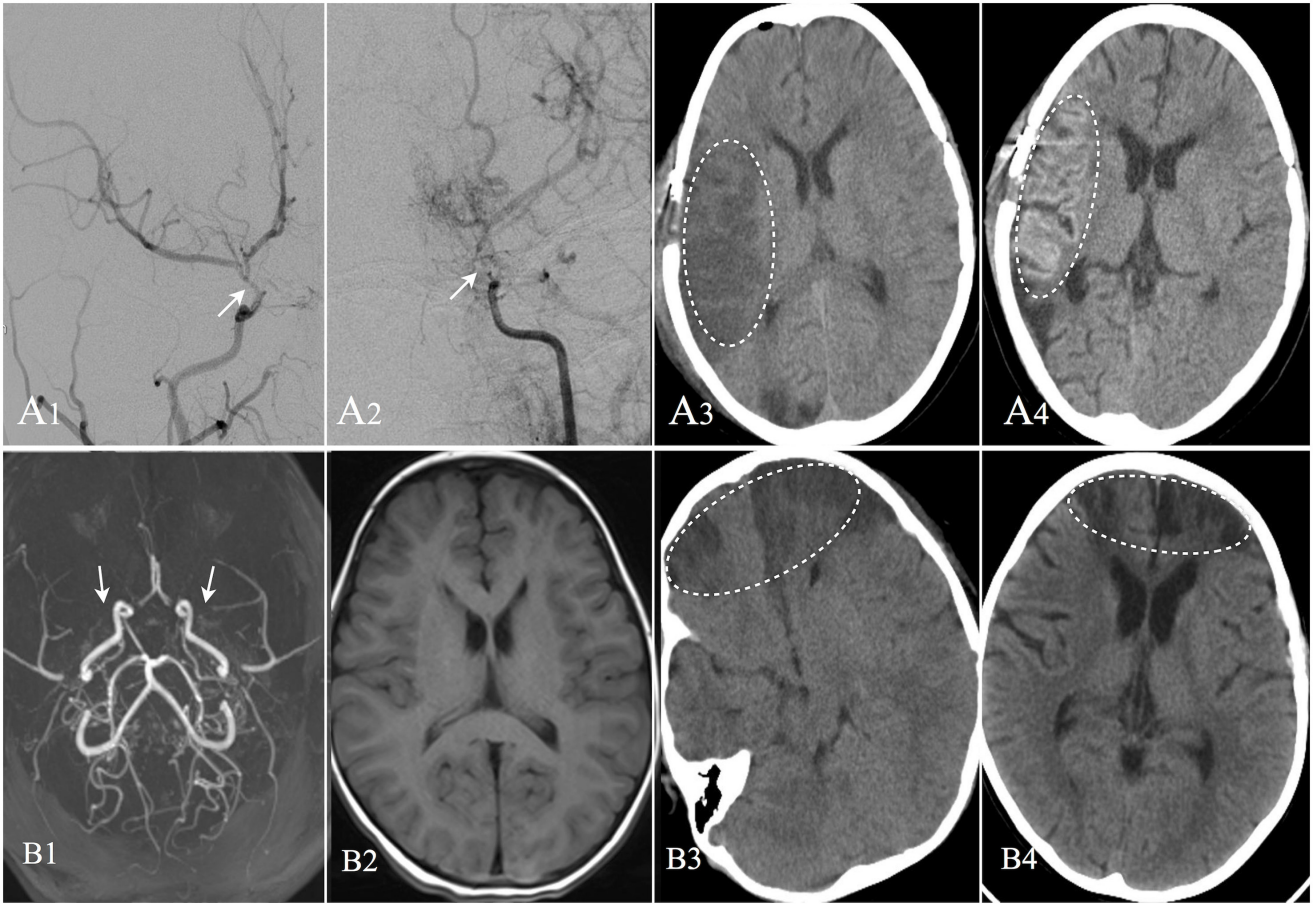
Two cases suffered postoperative operate-related infarction were showed. Patient A: 8 years old male, DSA confirmed moyamoya disease (A1,A2). CT image showed cerebral infarction occurred in the right operative region at the second day after EDAS operation (A3). CT performed at the 13th day after operation showed the infarction region with cortical laminar necrosis (A4). The patient was discharged with weakness in his left limbs; Patient B: 4 years old female, MRA confirmed moyamoya disease (B1). MRI image showed no remote cerebral infarction (B2). CT image showed cerebral infarction occurred in bilateral frontal lobes at the second day after EDAS operation (B3). CT performed at the 20th day after operation showed improvement in the infarction region (B4), and the patient was discharged with weakness in her upper right limb.
